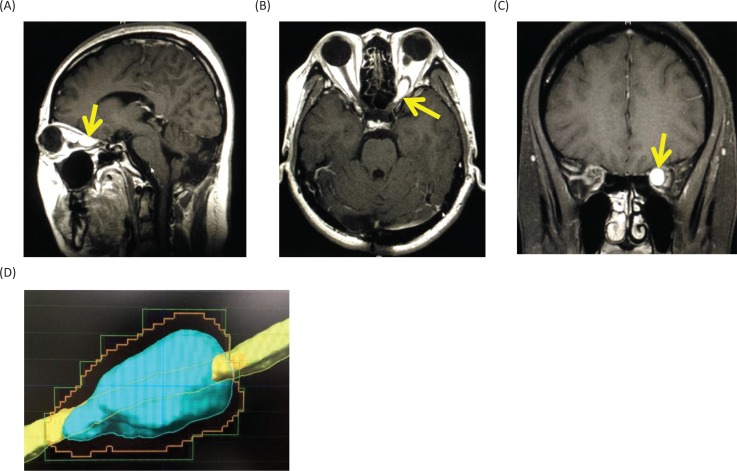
Magnetic resonance images before radiosurgery. (A) Gadolinium (Gd)-enhanced T1-weighted image, sagittal. (B) Gd-enhanced T1-weighted image, axial. (C) Gd-enhanced T1-weighted image, coronal. Yellow arrows show the tumor. (D) Three-dimensional planning MRI for radiosurgery. The light blue color indicates the tumor enveloping the optic nerve.
